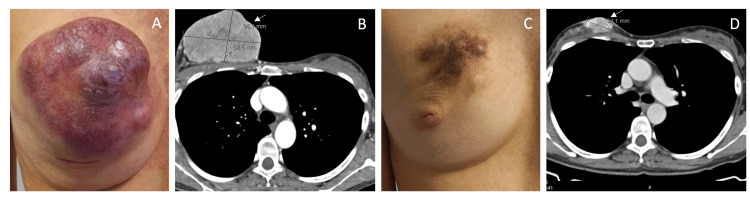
Right breast clinical and imaging findings, before and after neoadjuvant chemotherapy (A) Observation at diagnosis showing a violaceous and infiltrative mass occupying the upper quadrants of the right breast, consistent with cutaneous involvement. (B) Contrast-enhanced CT scan of the chest demonstrating a large right breast lesion measuring approximately 95 mm in its greatest dimension before treatment (arrow). (C) Marked clinical improvement after neoadjuvant chemotherapy (five months), with residual post-treatment pigmentation and skin retraction. (D) Post-chemotherapy CT scan showing a significant reduction of the primary lesion to approximately 34 mm (arrow).
